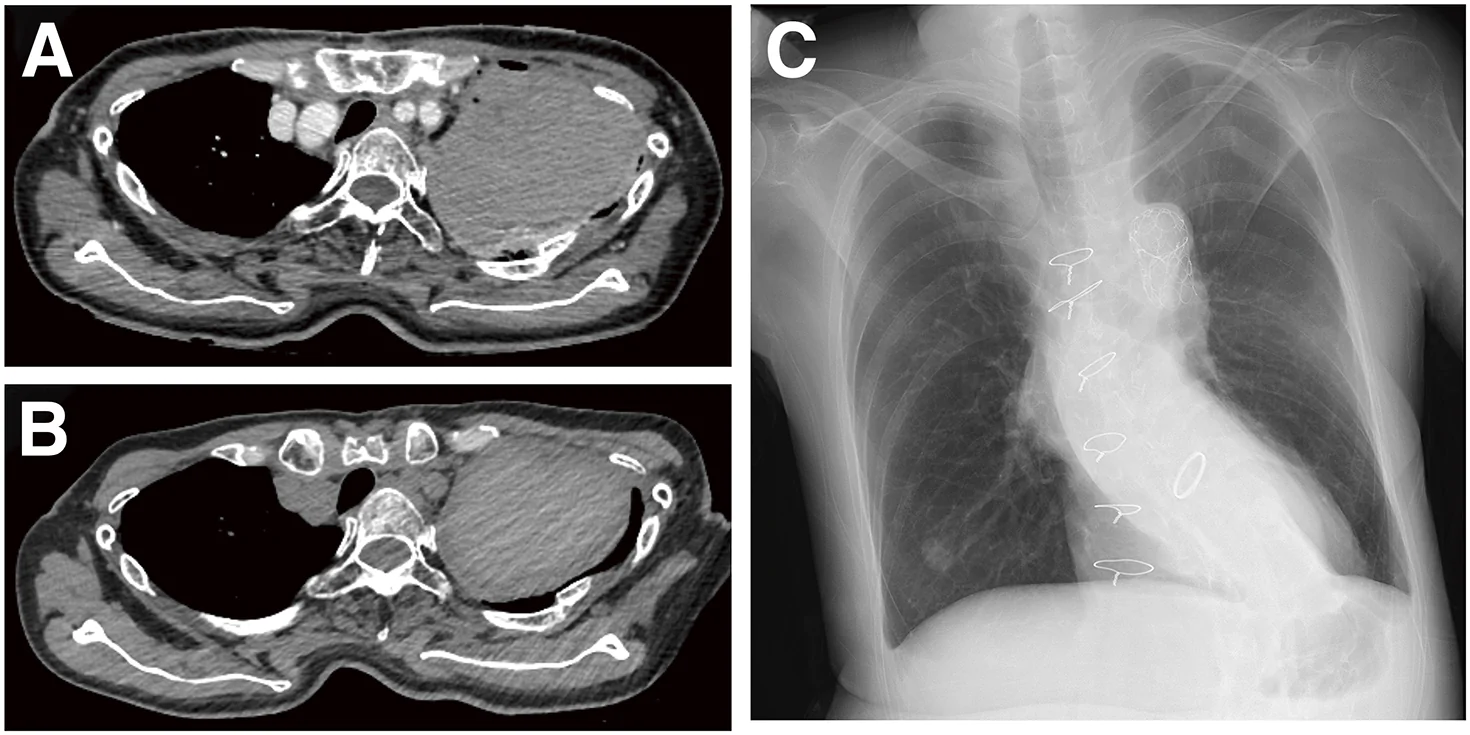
Follow-up imaging after embolization. (A) Contrast-enhanced CT performed 3 days after embolization showed no contrast extravasation or pseudoaneurysm. (B) Non-contrast CT performed 2 weeks after discharge demonstrated a reduction in the size of the hematoma. (C) Chest radiography at the 7-month follow-up visit demonstrated resolution of the hematoma.
